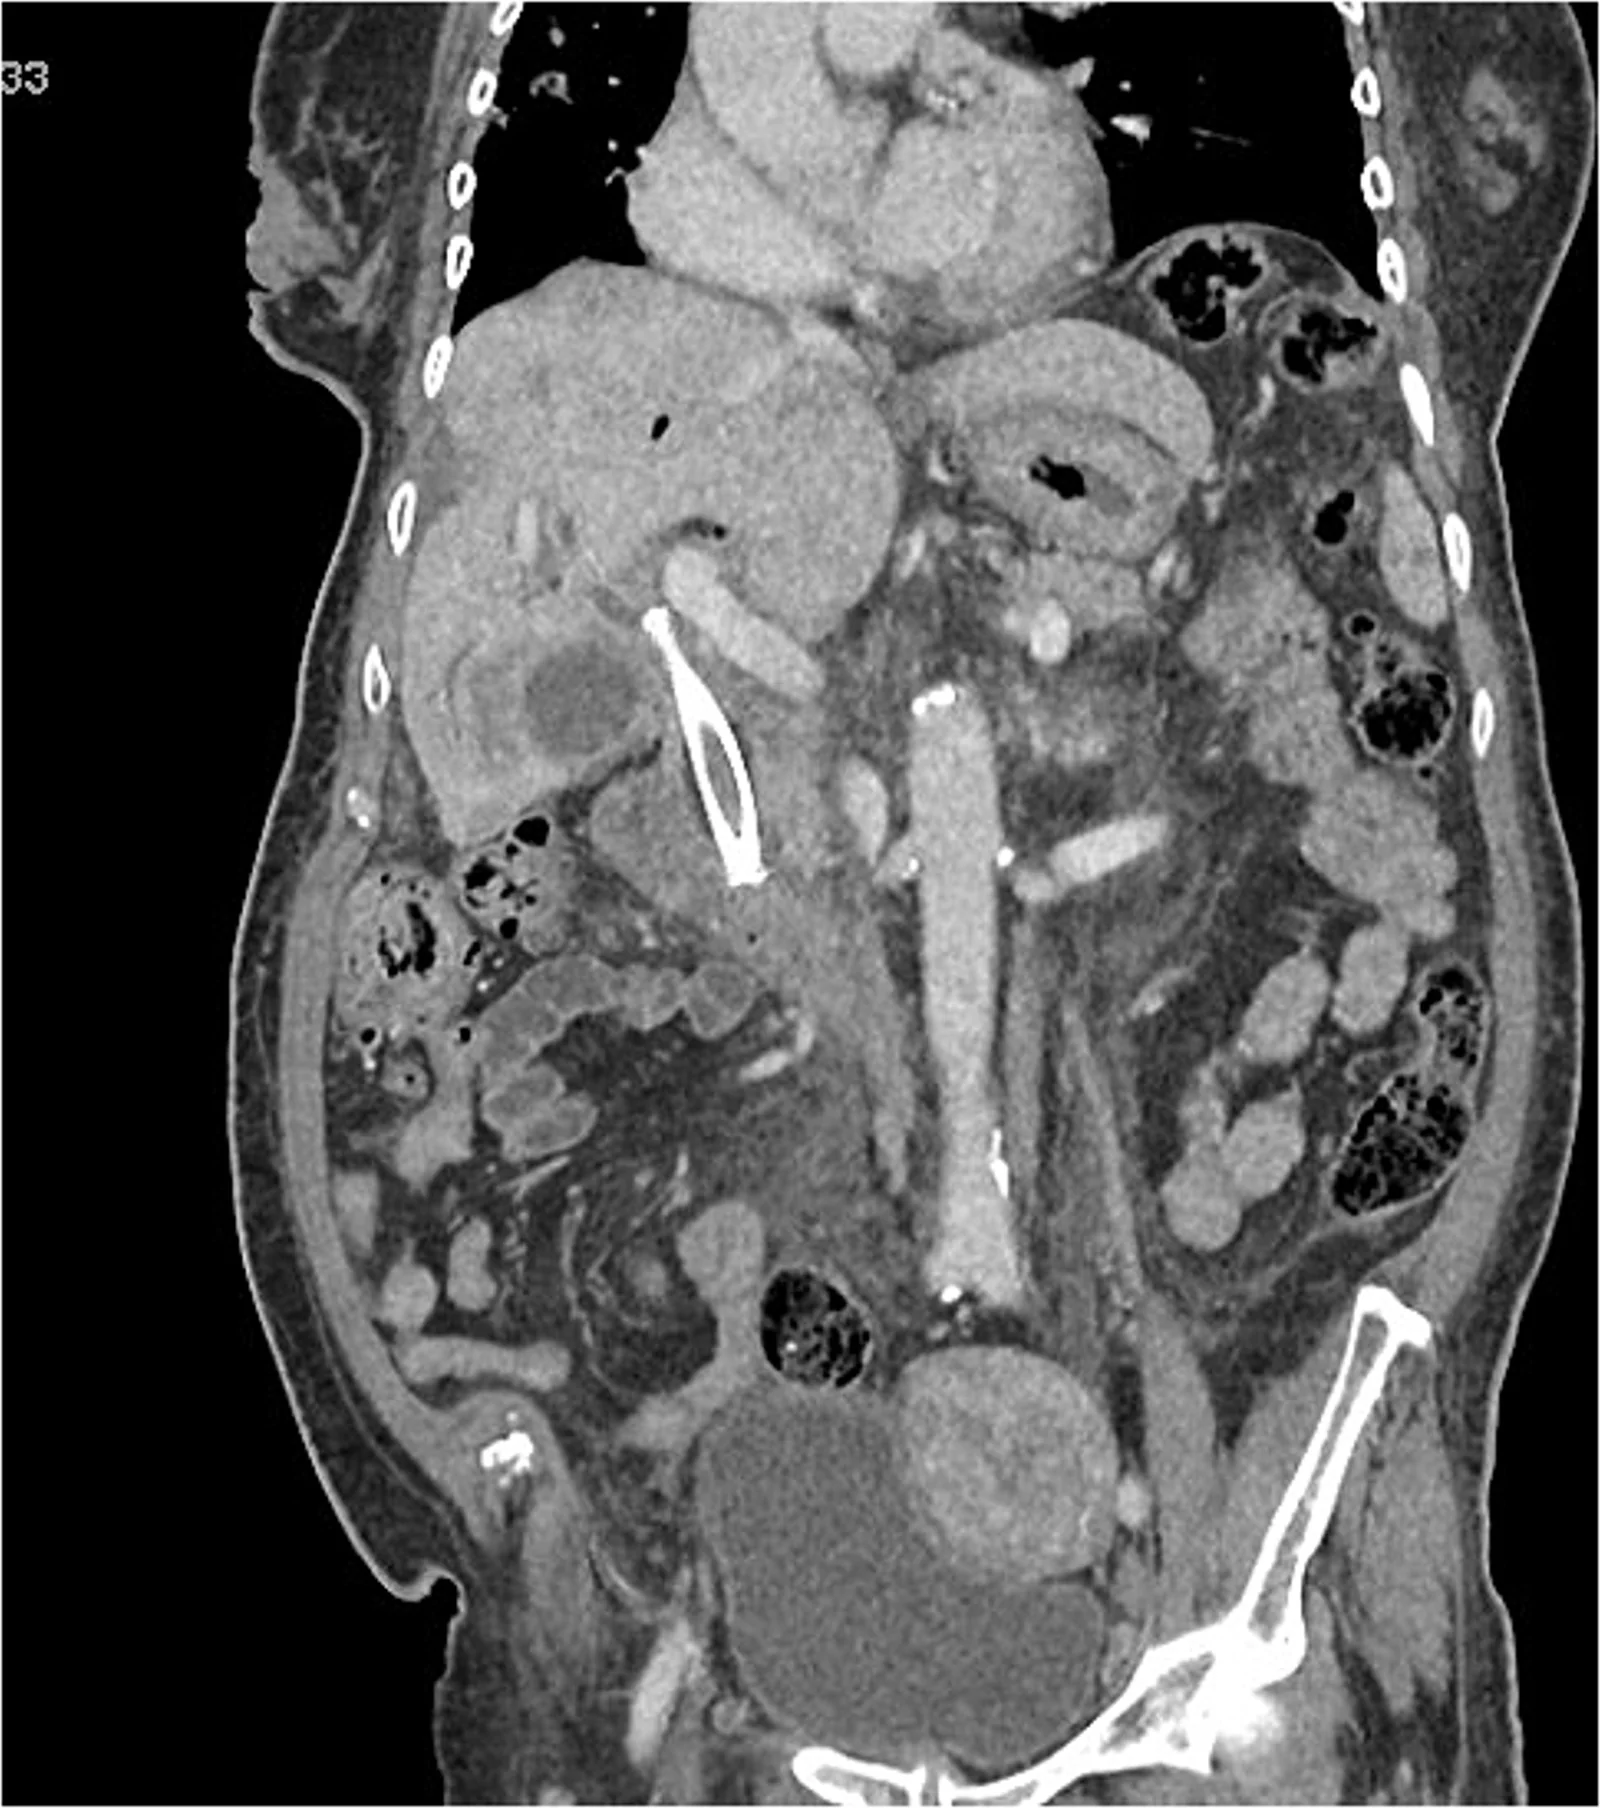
CT scan of the abdomen showing pneumobilia and an indwelling fully covered self-expanding metal stent in the biliary tree with maintained patency.
